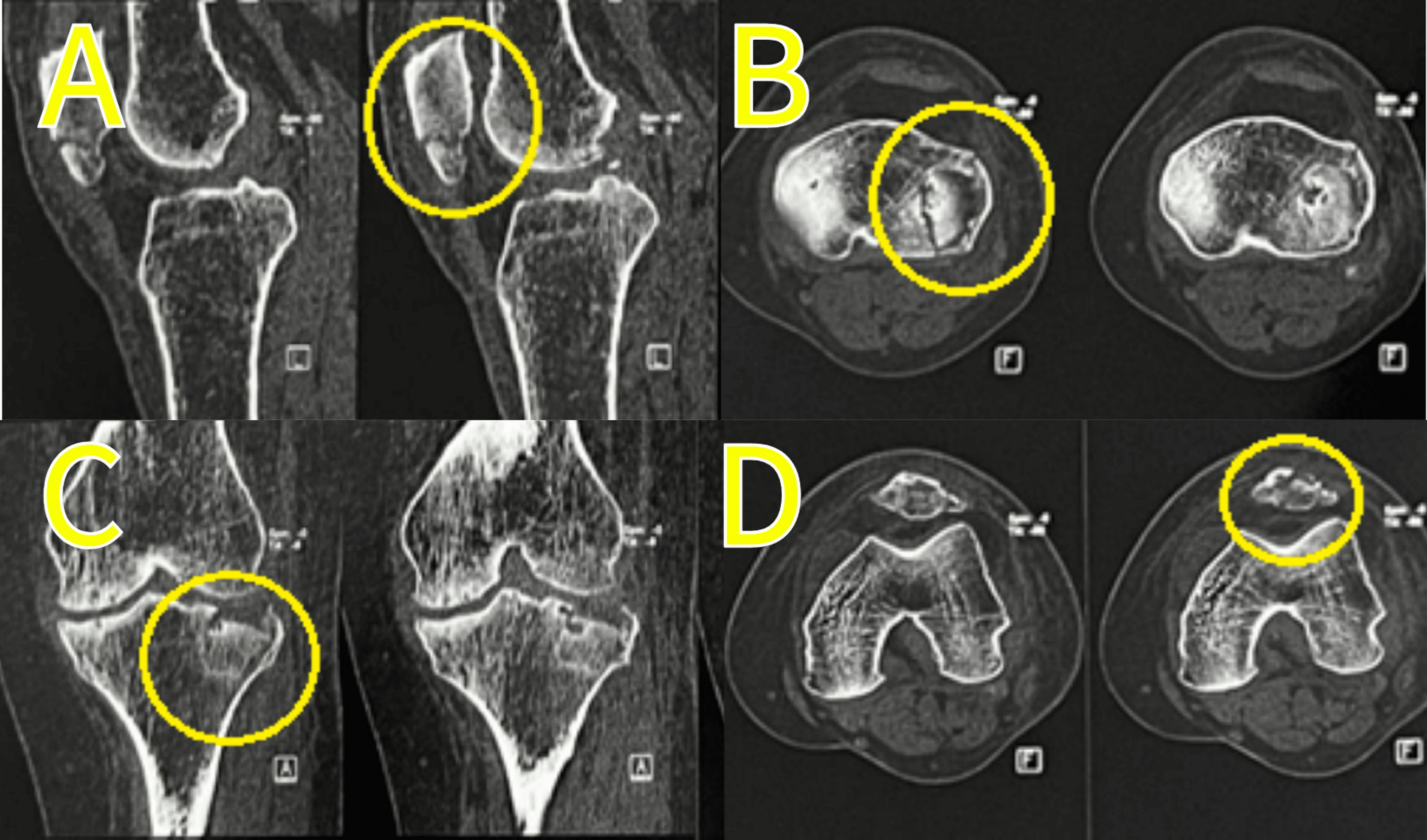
CT scan of the patella and proximal tibia (A) Sagittal view showing a lower pole of the patella fracture; (B) axial view of the proximal tibia showing a Schatzker type 3A fracture; (C) coronal view showing a split-depressed fracture of the proximal tibia; (D) axial view of the lower end of the patella showing a fracture
